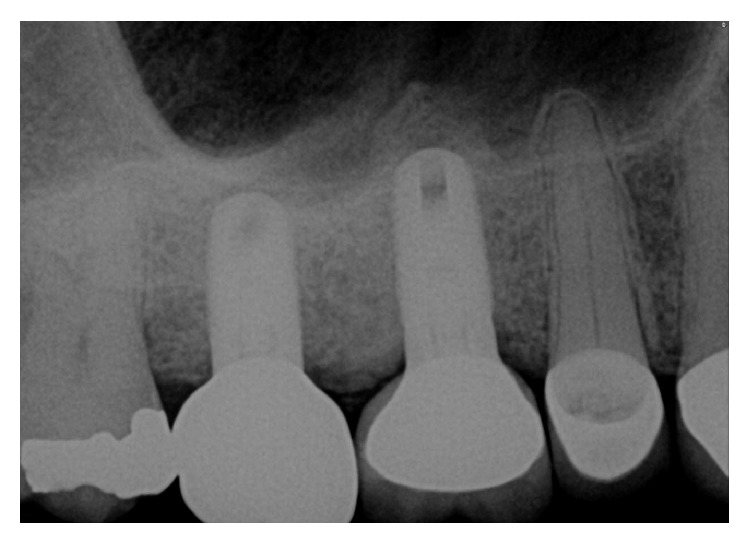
A periapical radiograph at 6 months postoperatively demonstrated an increased density around the implant apex.
